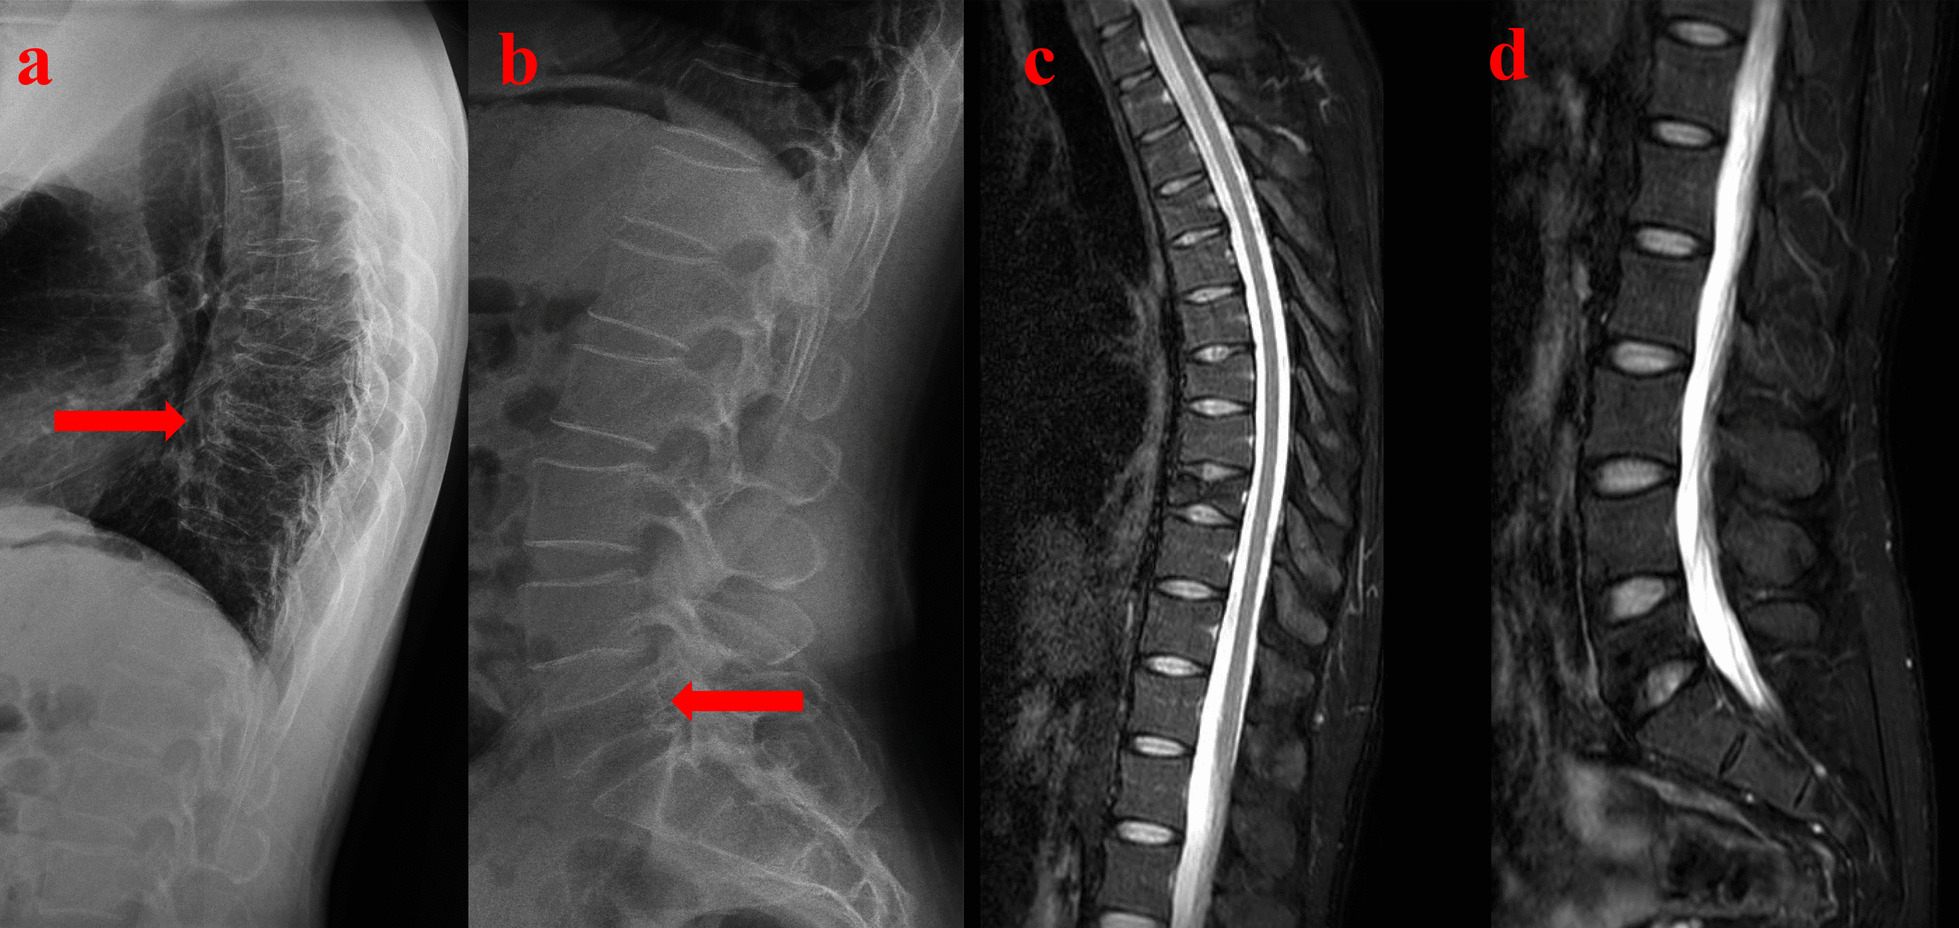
Radiology date of the patient at 3-year follow-up. a The lateral X-ray showed showed T9. b The lateral X-ray showed L5. c Magnetic resonance imaging (MRI) showed T9 VCFs. d MRI showed L5 VCFs. Arrows showed the T9 and L5 fracture
